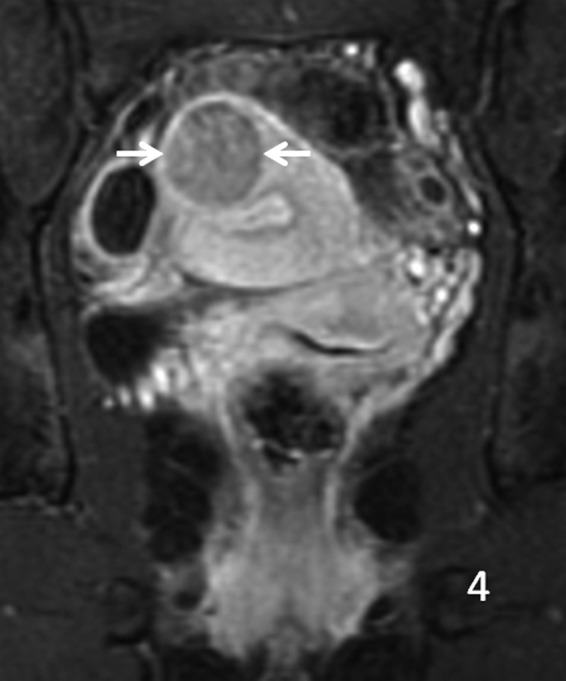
Coronal T1 MRI with contrast, arrows showing mild signal enhancement.
